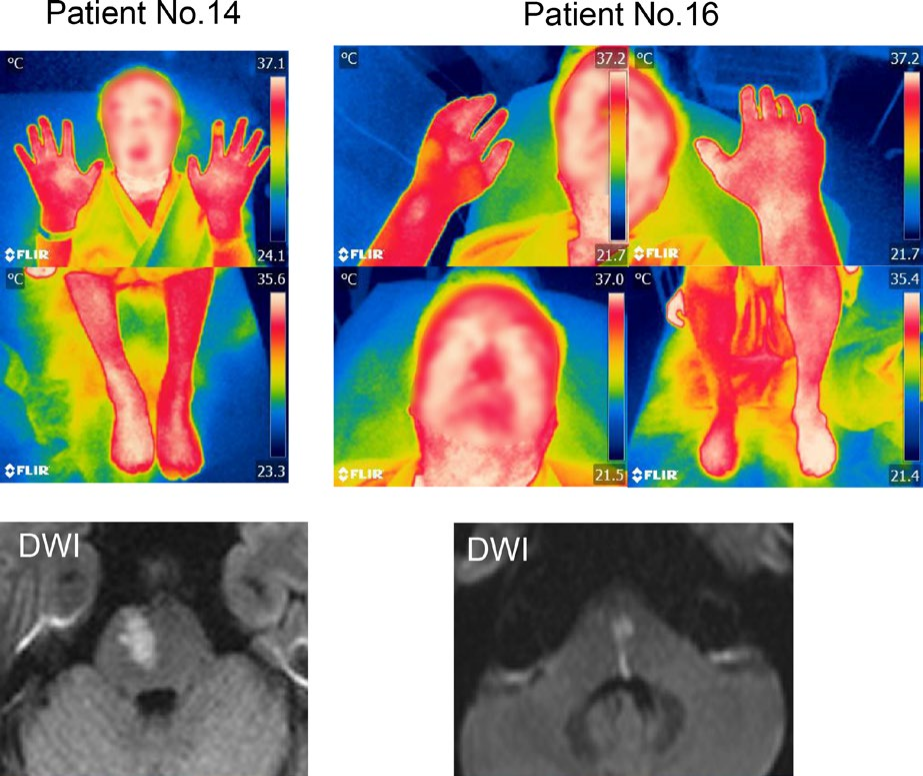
Picture taken by thermographic camera and MRI findings in pontine infarction patients with laterality of BST. Two patients with lower pontine infarction showed the laterality of BST only at one or two of their extremities (The patients’ faces are obscured by a mosaic for personal information protection)
